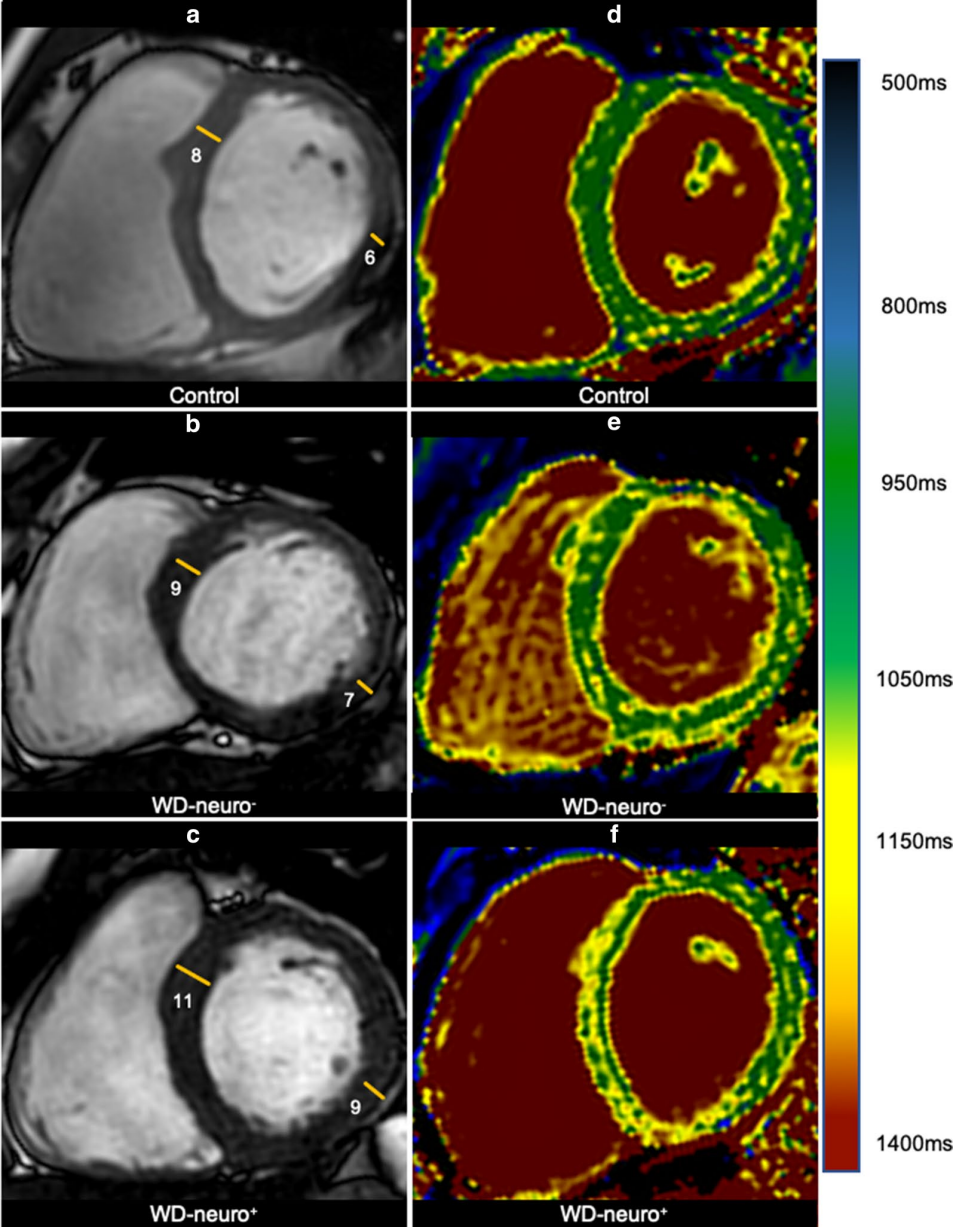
Cine images and native T1 MOLLI in patients with Wilson Disease (WD) with and without neurological symptoms. Images of left ventricular in Wilson Disease (WD) patients: controls (a) (male, septum 8 mm, lateral wall 6 mm), WD patients without neurological symptoms (WD-neuro−) (b) (male, septum 9 mm, lateral wall 7 mm) and WD patients with neurological symptoms (WD-neuro+) (male, septum 11 mm, lateral wall 9 mm) (c). Images of native T1 times in controls (d) (female, global native T1 times 1014 ms), WD-neuro− (e) (female, global native T1 times 1055 ms) and WD-neuro+ (f) (male, global native T1 times 1070 ms). Colors (1.5 T MRI); color scale displays T1 values (ms)
